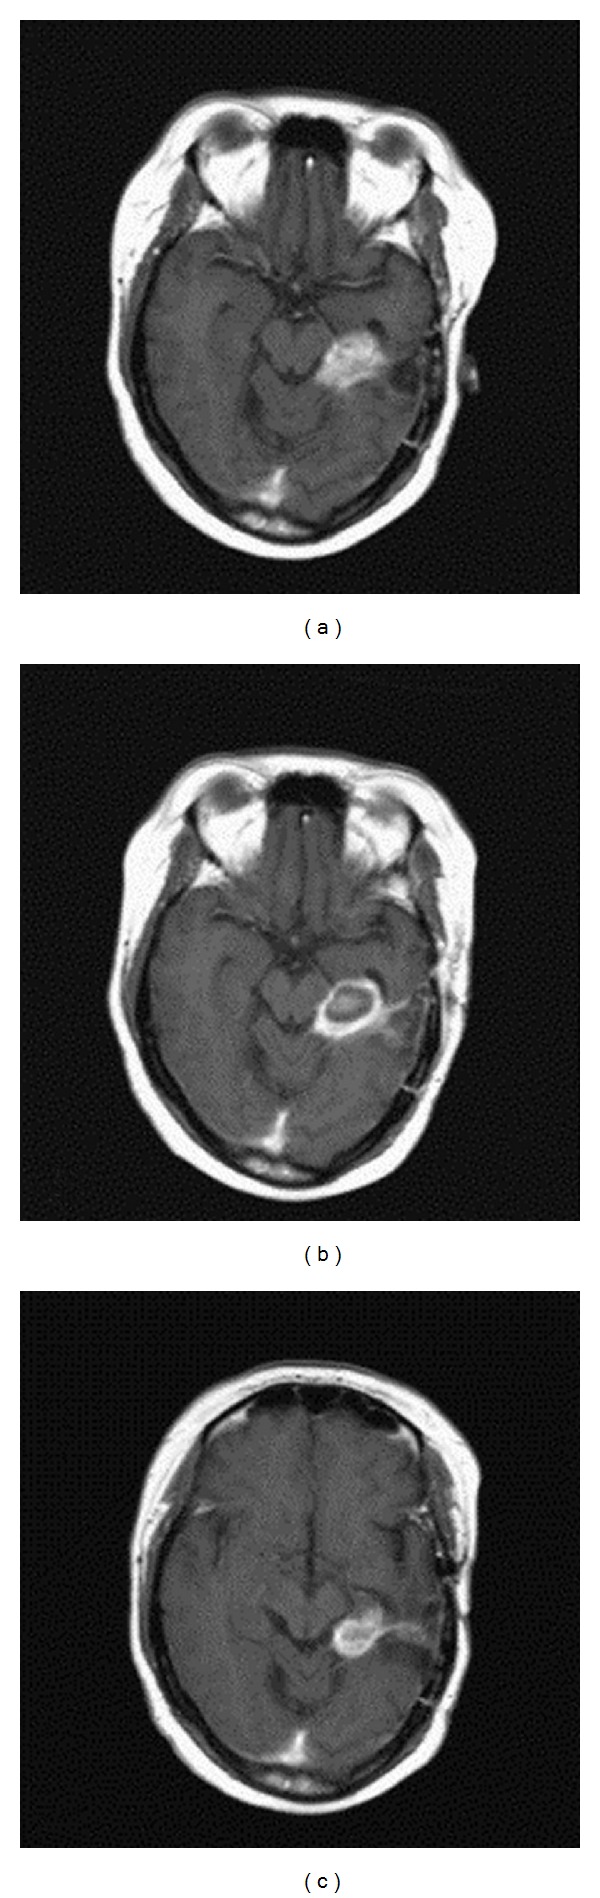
MR imaging of treatment progression of a recurrent GBM patient [4]: (a) Preoperative state and (b) 24 hours after LITT. A characteristic “ring” can be seen, indicating that necrosis has been achieved in the center of the tumor mass. (c) 17 months after LITT, tumor volume is reduced.
